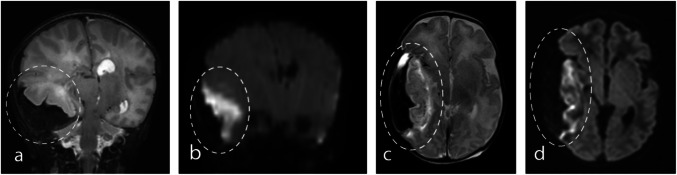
Neonatal subpial hemorrhages with Yin-yang sign. Brain MRI in a 1-day-old term male with apneic events and seizures (a-b). Coronal T2-weighted image (a) shows low signal intensity corresponding to subpial hemorrhage splaying underlying sulcus, and DWI (b) shows a bright underlying cerebral cortex with cytotoxic edema of temporal lobe cortical ribbon indicating underlying infarcted brain parenchyma (dotted circles). Brain MRI in a 6-day-old term male with forceps delivery and apnea (c-d). Axial T2-weighted image (c) and DWI (d) show the combination of a dark subpial hemorrhage and a bright underlying cerebral cortex (extensive cytotoxic edema) resembling the yin-yang symbol ( dotted circles)
